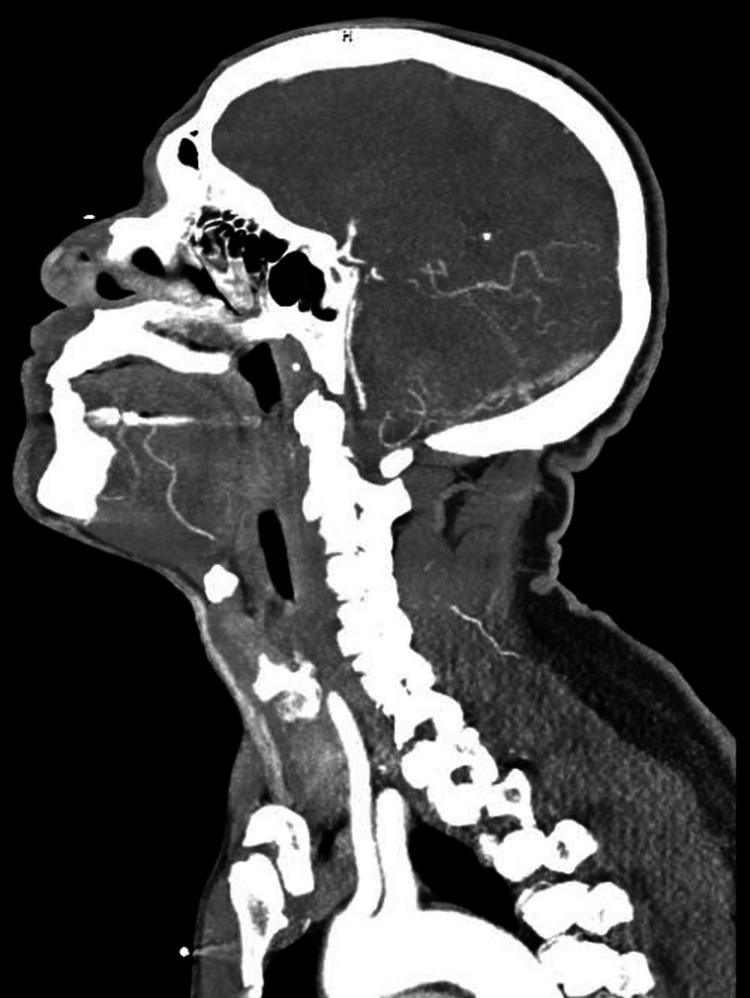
Sagittal view of the CTA of head and neck. CTA = computed tomography angiography
